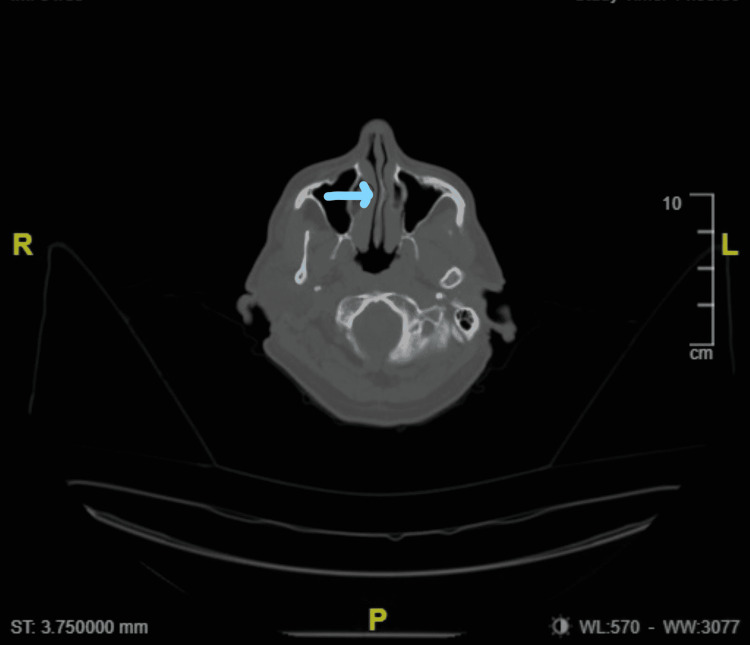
Computerized tomography (CT) of the head Coronal non-contrast head CT image showing left-sided nasal bone spur (blue arrow).
